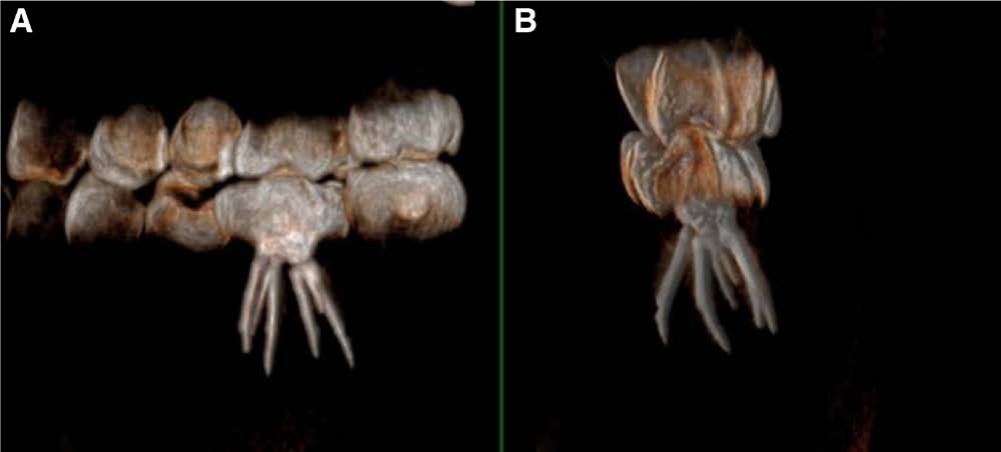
Three-dimensional reconstruction of the CBCT images of tooth 36. (A) Coronal view. (B) Sagittal view. CBCT = cone-beam computed tomography.
